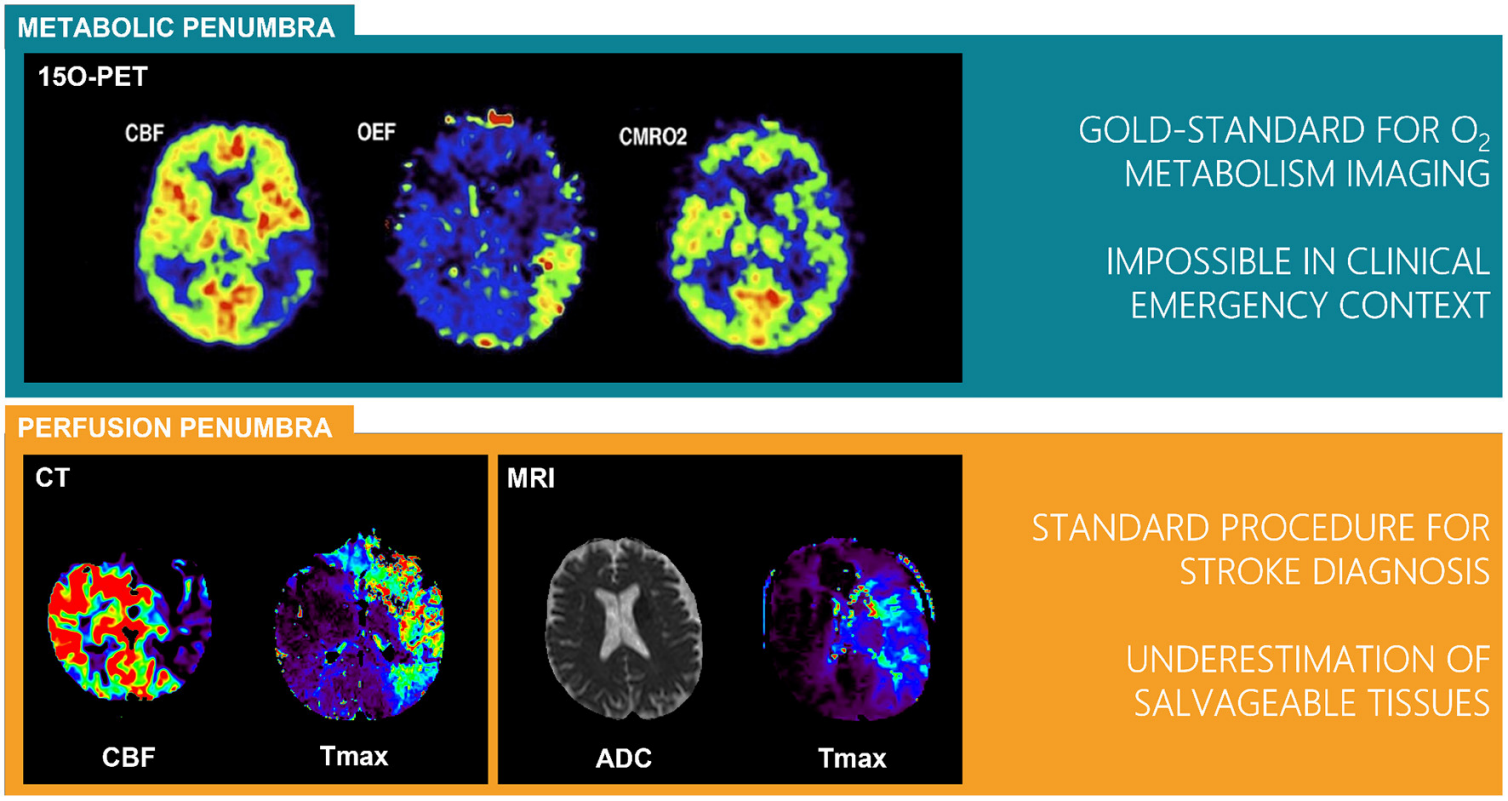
Definitions of perfusion and metabolic penumbra from the gold standard [^15^O]-Positron Emission Tomography (PET) to the widely distributed Computed Tomography (CT) and Magnetic Resonance Imaging (MRI) perfusion imaging. PET images published in JNM. Heiss WD. Radionuclide imaging in ischemic stroke. *J Nucl Med*. (2014) 55:1831–41. SNMMI.; © CT and MRI images from Olea Medical. CBF, cerebral blood flow; CMRO_2_, cerebral metabolic rate of oxygen; OEF, oxygen extraction fraction; ADC, apparent diffusion coefficient; Tmax, time to maximum of residual function.
